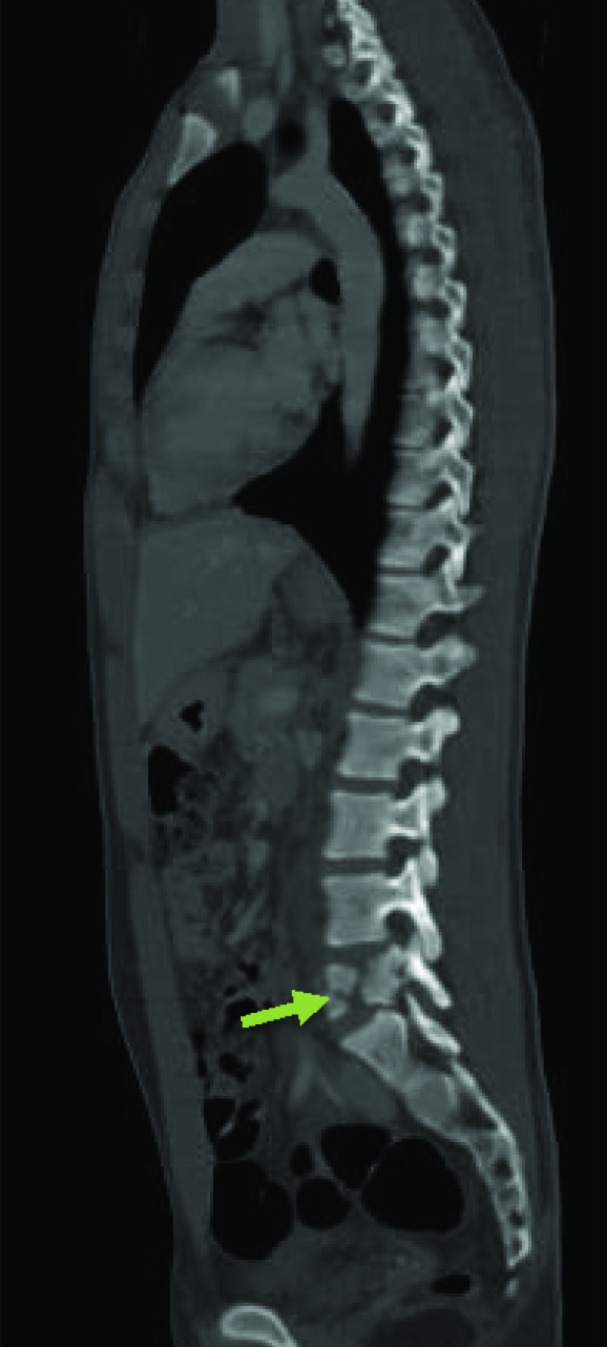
Sagittal Computed Tomography (CT) scan of the whole spine showing burst fracture at L5 (arrow) with canal compromise (Magerl A3)
